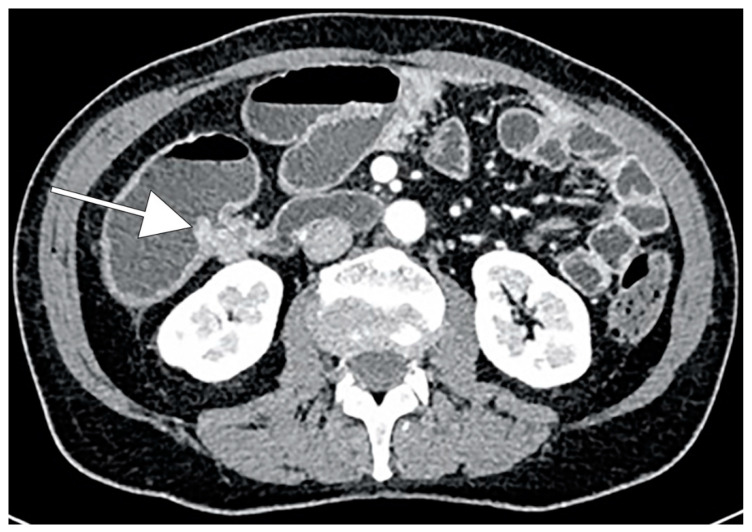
CT scan demonstrating wall thickening colorectal cancer (CRC) of the right colon (white arrow).
